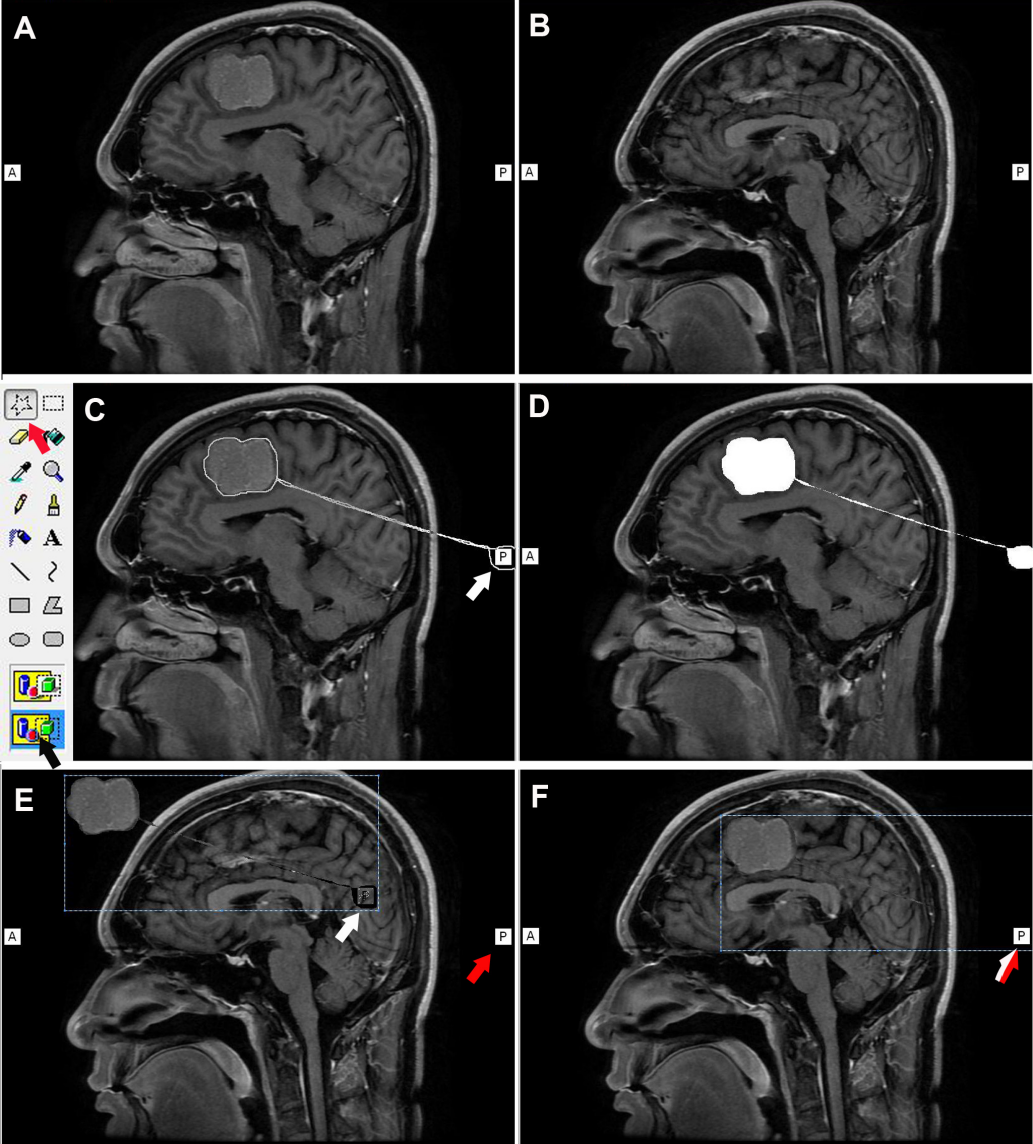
Preprocessing of the MR images. (A) A sagittal slice showing the maximal tumor boundary is selected as the first image. (B) A mid-sagittal slice is selected as the second image. (C) In MS Paint, the “Free-Form Select” tool (red arrow) and “Transparent Select” tool (black) are chosen. The tumor and the “P” label (white arrow) are selected together. (D) The selected tumor and the “P” label are cut out together. (E) The selected tumor and “P” label (white arrow) are pasted into the mid-sagittal slice. The red arrow indicates the corresponding “P” label in the mid-sagittal slice. (F) By making the two “P” labels overlap (half white and half red arrow), the projection of the tumor on the mid-sagittal slice is correctly depicted.
